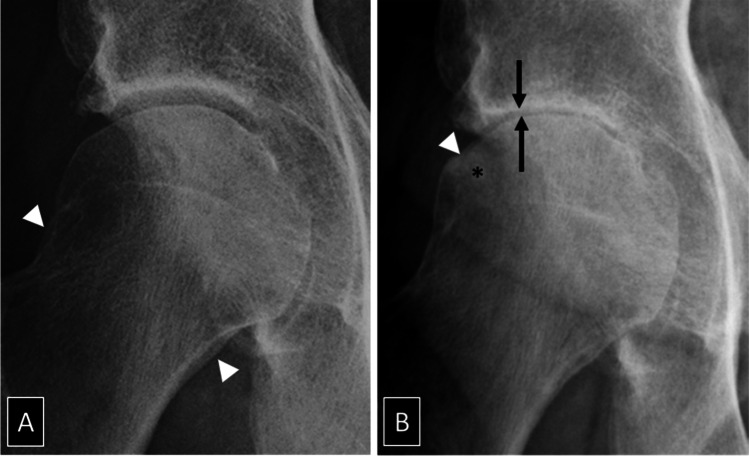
65-year-old man with rapidly destructive osteoarthritis. A AP radiograph of the right hip obtained at onset of symptoms shows early osteophytes (arrowheads) but no joint space narrowing. B AP radiograph obtained 3 months later shows complete joint space narrowing (arrows) with deformity of the femoral head (arrowhead) and subchondral sclerosis (asterisk).
